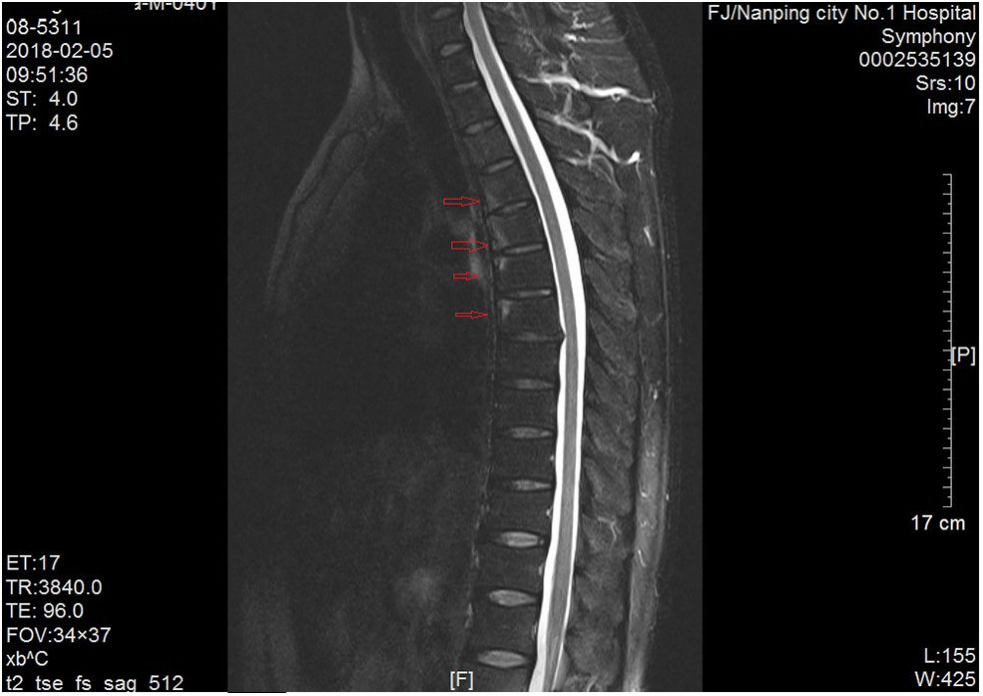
MRI examination of thoracic vertebrae. Abnormal signal of T4–T7 was indicated by a red arrow.
